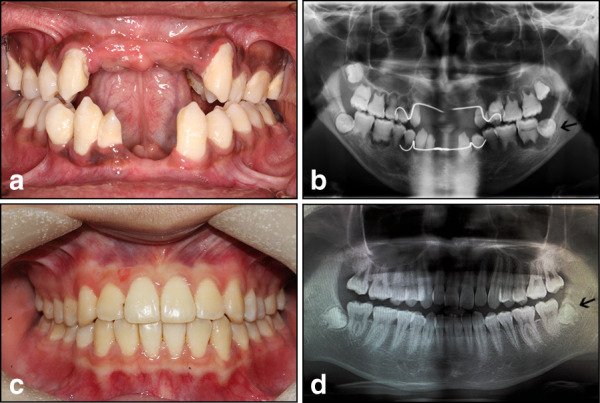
Intraoral images and panoramic radiographs from the individuals. a Intraoral image of the patient with DD-I. b Panoramic radiograph of the patient with DD-I. c Intraoral photo of the healthy control. d Panoramic radiograph of the healthy control. The apical development of the third molars of the DD-I patient and the healthy control was not yet completed (black arrow)
